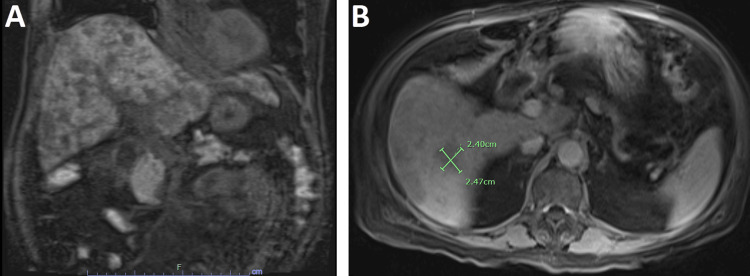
MRI abdomen with and without contrast with MRCP A: Coronal section shows nodular liver with cirrhotic morphology and normal gallbladder, biliary tree, and pancreas. B: The transverse section shows multiple hypoenhancing lesions such as the 2.4 x 2.47 cm on the image. MRCP: magnetic resonance cholangiopancreatography
